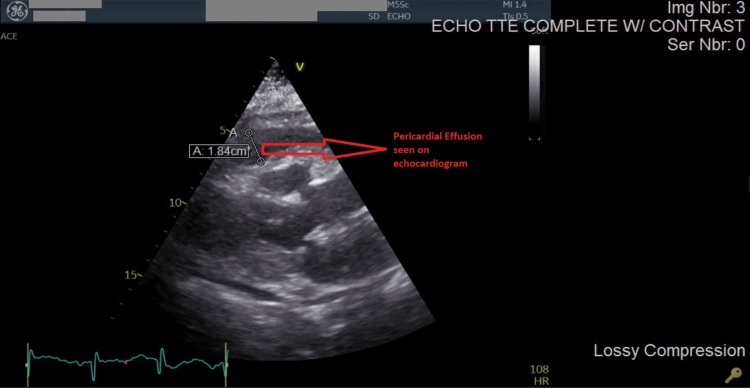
Pericardial effusion on echocardiographic imaging
